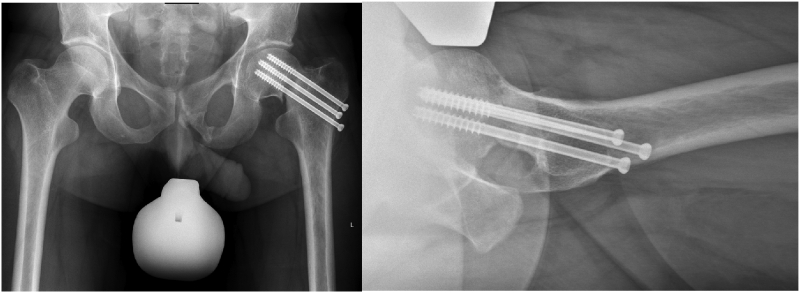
AP pelvis and cross-table lateral hip radiographs show no significant fracture union following placement of three partially threaded, 7.3 mm cannulated screws.
